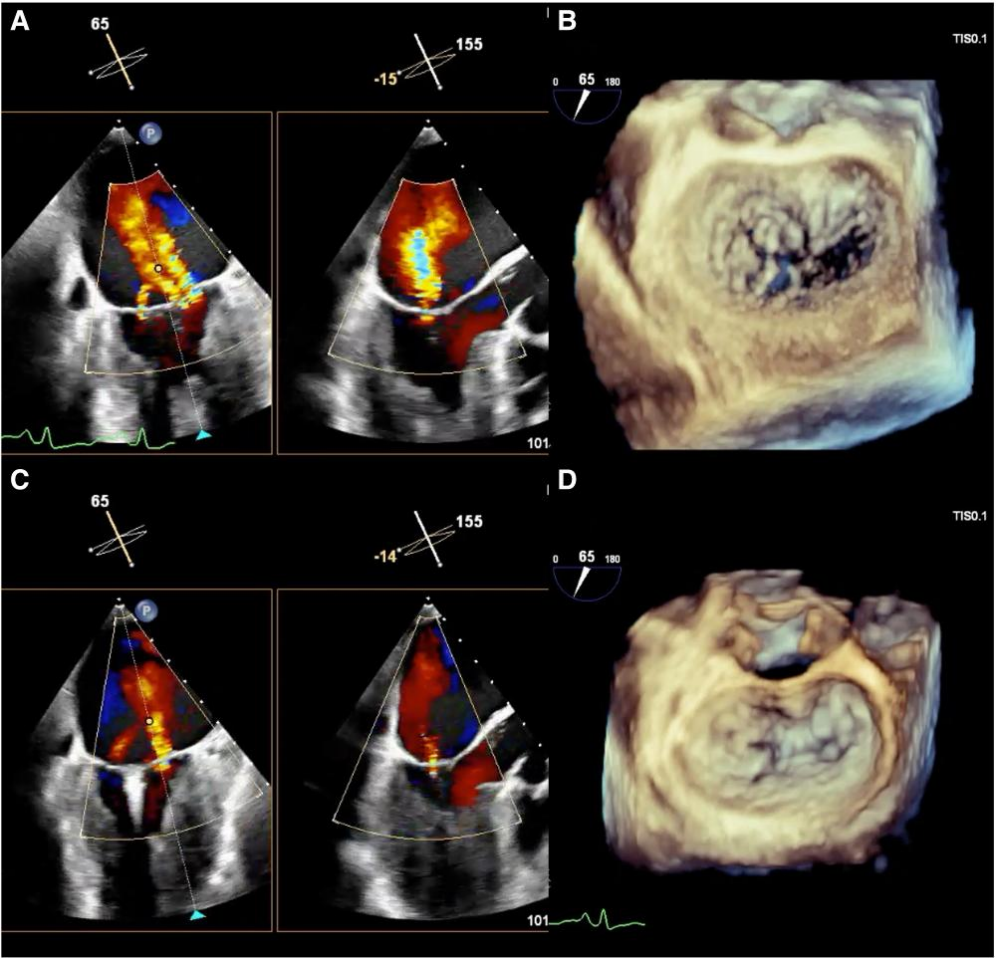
Transoesophageal echocardiographic findings during a transcatheter mitral valve repair procedure. An MR jet is identified in the A2-P2 lateral indentation and the valve leaflets are detached (*A* and *B*). NTW clip at A2-P2 centre is implanted due to thickening of the subvalvular myocardium at A2-P2 lateral (*C* and *D*). MR, mitral regurgitation.
